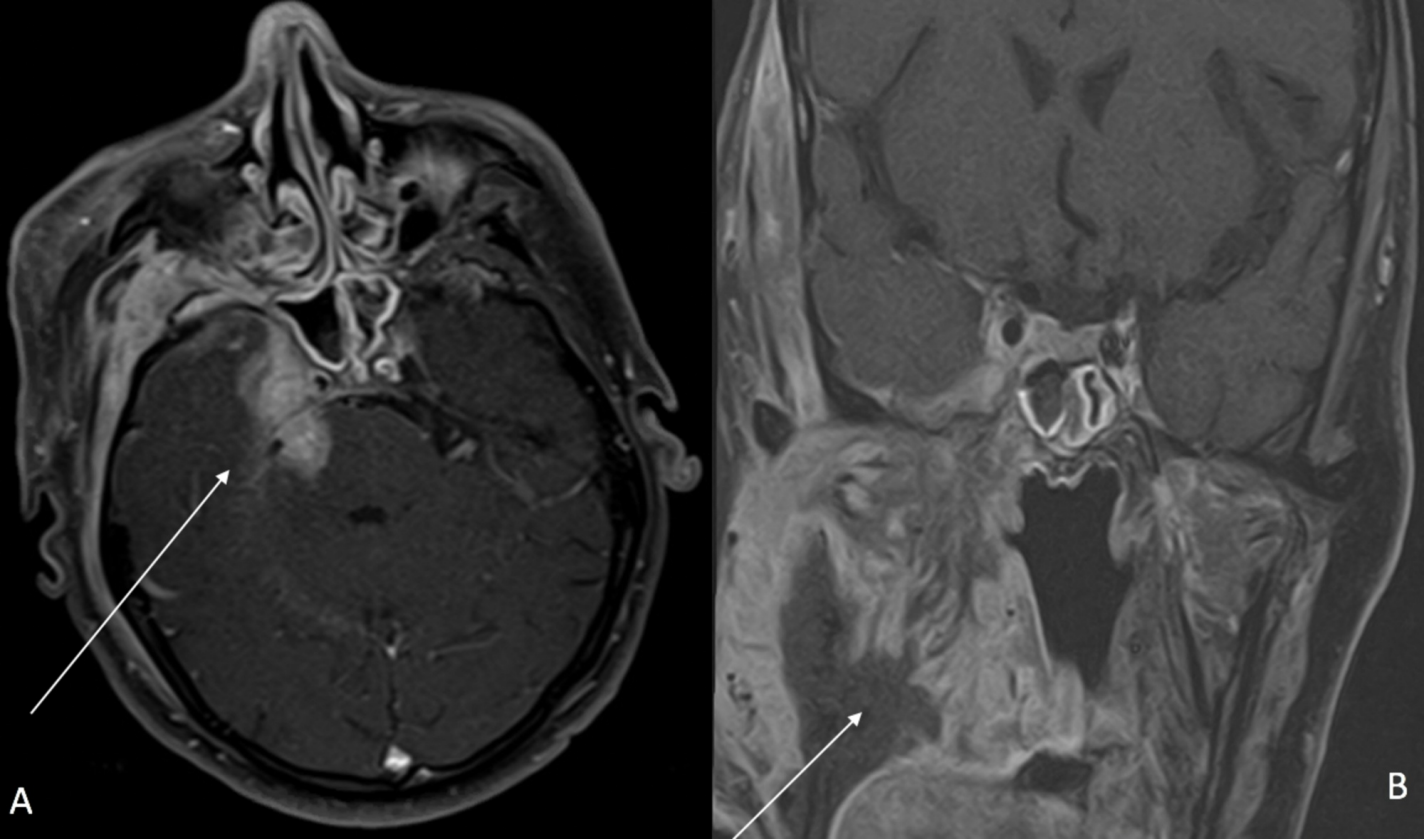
MRI brain and neck with contrast four months after pazopanib therapy The arrow in pane A (axial view) highlights the increased intracranial burden of disease with involvement of right Meckel's cave, right cavernous sinus and frank invasion into the ventral pons. The arrow in pane B (coronal view) points to the mild decrease in tumor burden extracranially especially in the right infrazygomatic masticator space and tumor necrosis.
